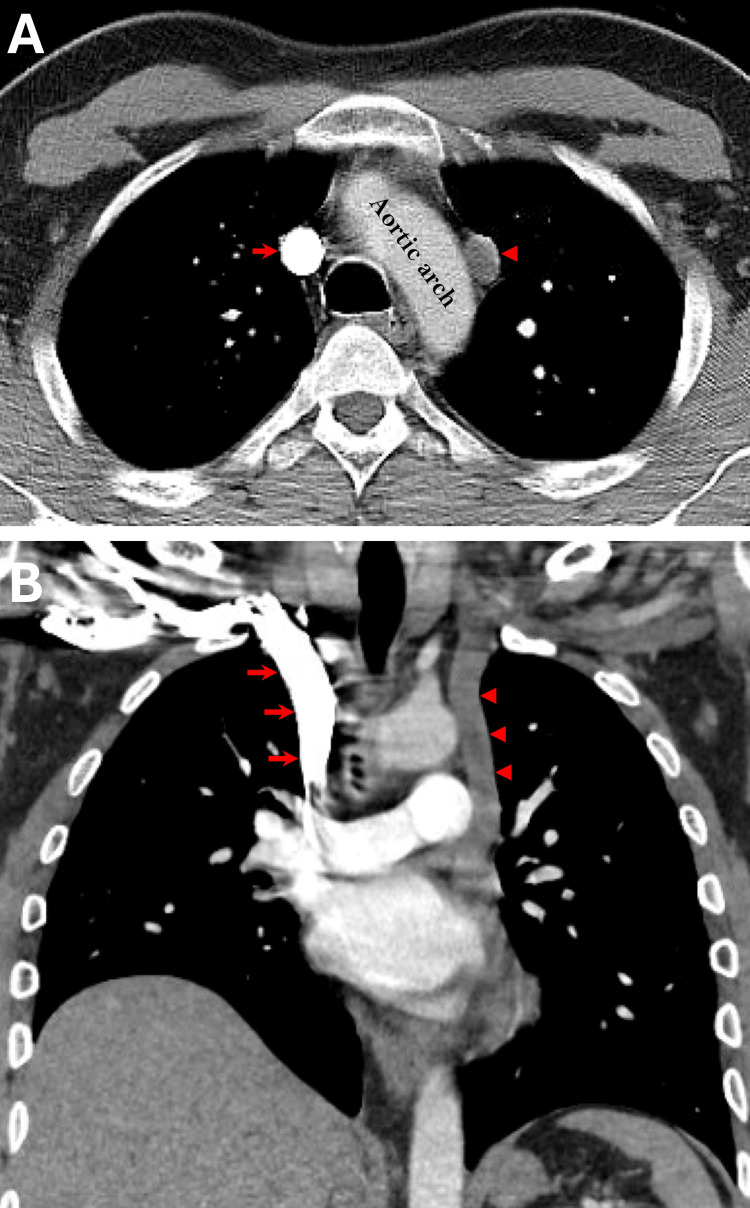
Computed tomography Axial (A) and coronal (B) images from a contrast-enhanced computed tomography scan of the chest demonstrate a tubular structure on the left side of the mediastinum (red arrowheads), with a parallel course to the normal right-sided superior vena cava (red arrows), consistent with the left-sided component of a duplicated superior vena cava. Note the dense opacification of the right-sided SVC due to injection of the intravenous contrast via a right upper extremity vein in this patient. The left-sided SVC contains unopacified venous blood returning from the left upper extremity. The aortic arch is partially opacified on this scan, acquired during an early phase of the contrast administration. SVC, superior vena cava
